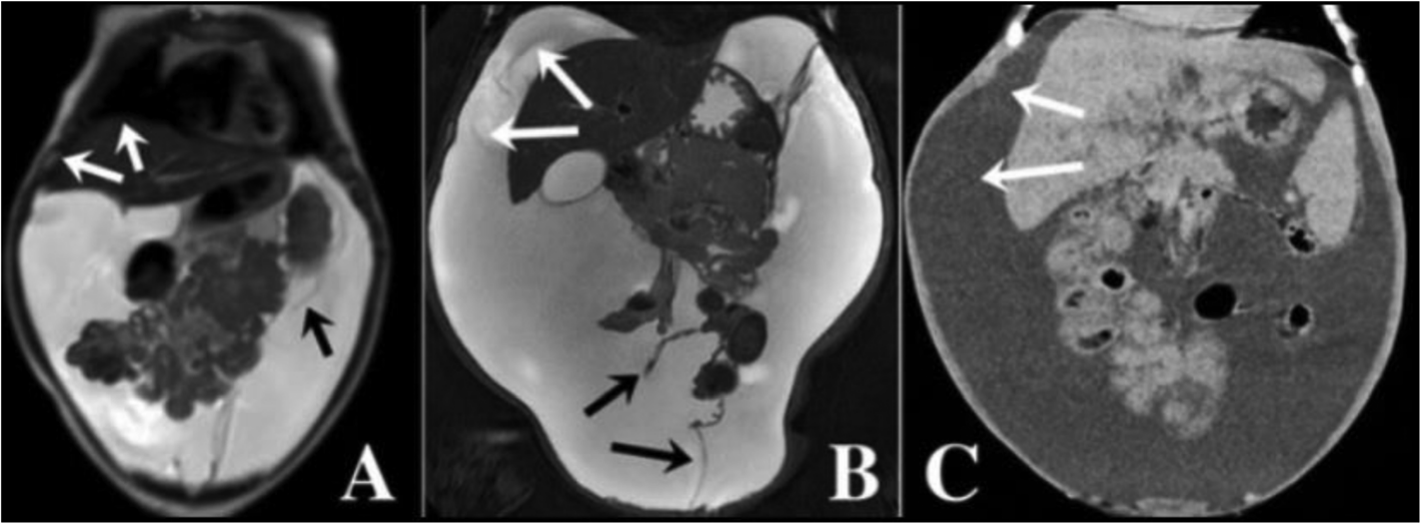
Radiological signs evoking pseudo-ascites. a Initial MRI of the patient: Coronal aspect showing distribution of the abdominal fluid and the sparing of the hepatodiaphragmatic space. Note the centralization of the bowel and the confusing floating aspect of the bowel loops, and the partial septae. b MRI performed at a later stage in the patient: Coronal aspect showing an increased amount of fluid that distributes in the whole cavity and do not spare the hepato-diaphragmatic space. c as a comparison, CT coronal aspect of free massive ascites in a child with decompensated biliary cirrhosis. (White arrows pointing at the hepato-diaphragmatic space – Black arrows pointing at partial septae)
